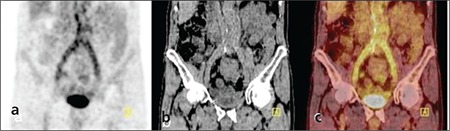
Coronal PET (a), CT (b) and PET/CT fusion (c) images
